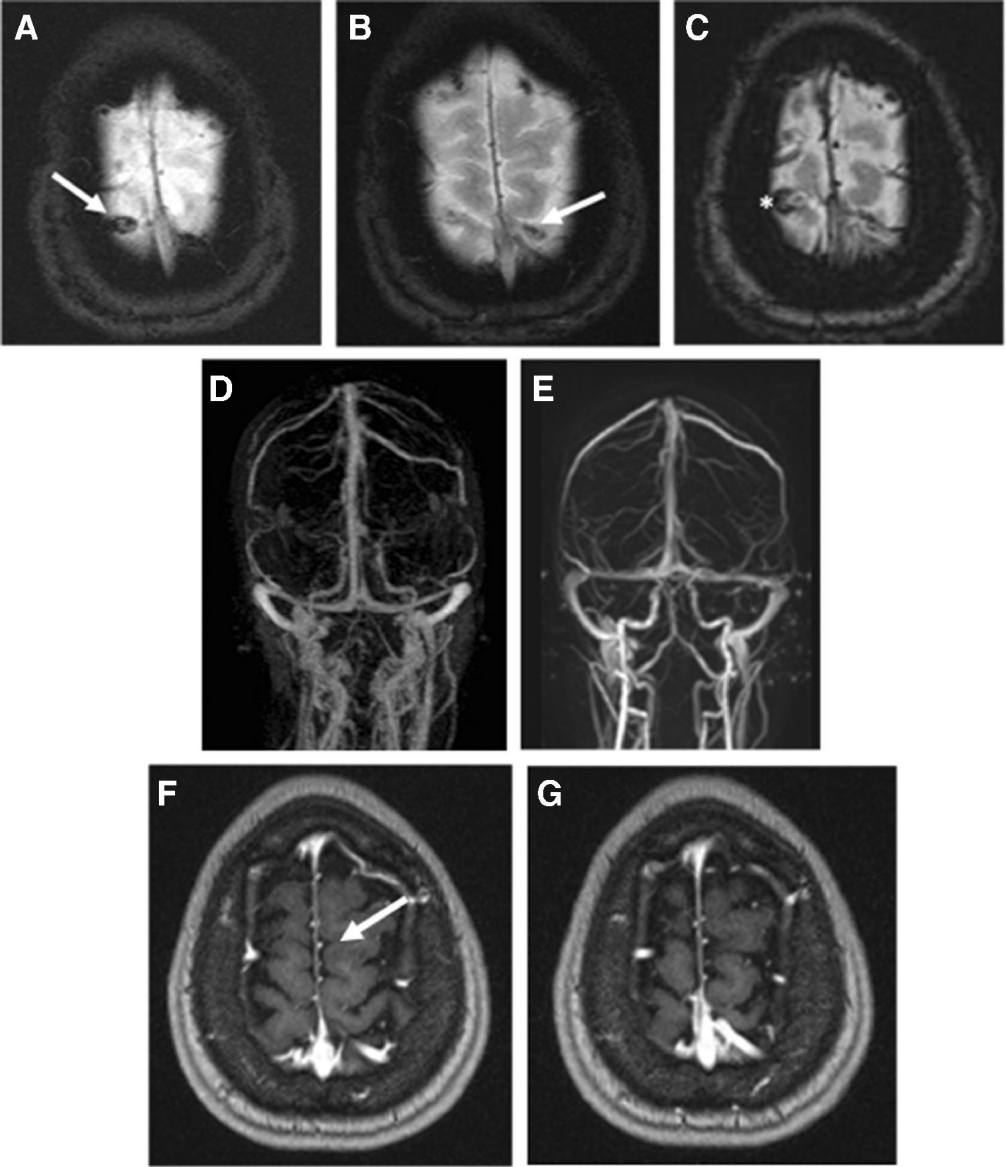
Thrombosis in the area of the cerebral veins bifrontal and bioccipital. A, B Low signal vascular courses in T2*-weighted imaging (arrows). C Blooming sign (asterisk) in susceptibility-weighted imaging (SWI). MRA with absent signaling of the bridging veins on the right (asterisk) in unenhanced PC-MRA (D) and CE-MRA (E). F, G Multiplanar 3D T1-weighted CE imaging demonstrates lack of contrast of thrombosed bridging veins (arrow)
